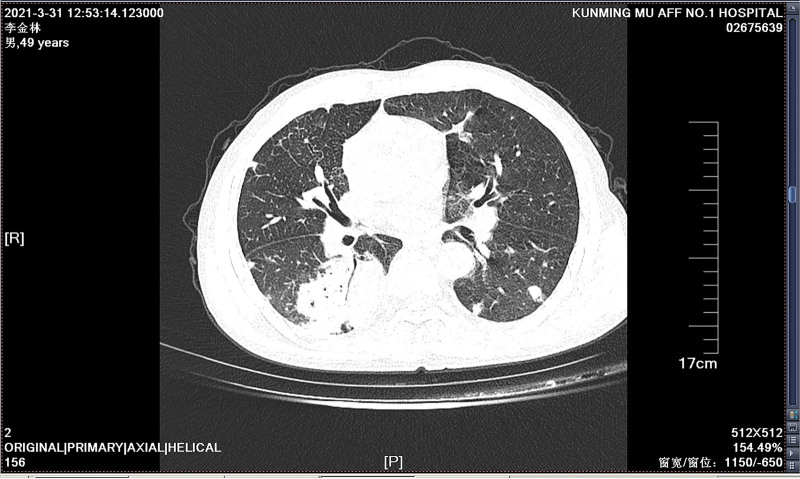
Chest CT on March 31, 2021 showed that large sheet consolidation of the upper and lower lobes of the right lung, bronchial air signs inside, multiple nodular high-density shadows in both lungs, and no definite enlarged lymph node shadows in the mediastinum. CT = computed tomography.
